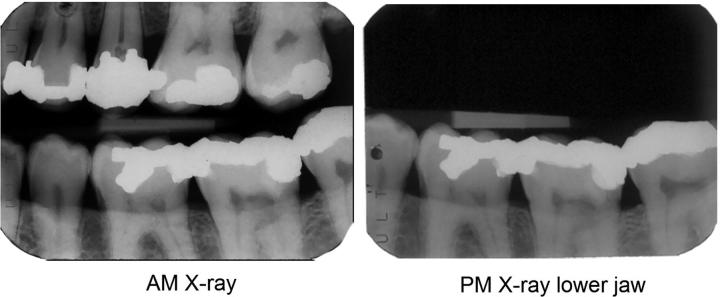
X-ray image comparison. The bright white areas in the tooth crowns represent the unique shapes of metal amalgam fillings. The chances of getting such a level of similarity between the teeth, filling shapes and bone levels in two individuals chosen at random would be exceedingly small, so it is reasonable to state that these images originate from the same person. (Image courtesy Health Support Queensland Forensic and Scientific Services (HSQ FSS) and the Queensland State Coroner, with permission).
